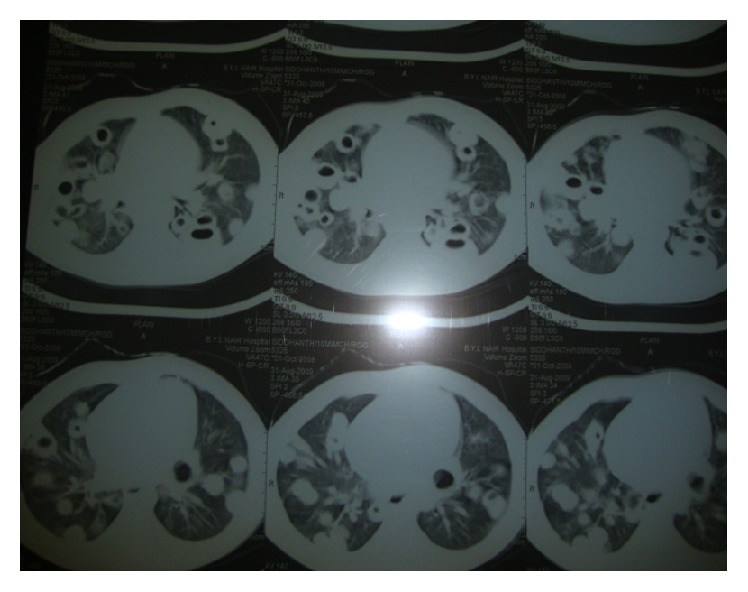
Computed tomography showing bilateral cavitary nodules and emboli.
